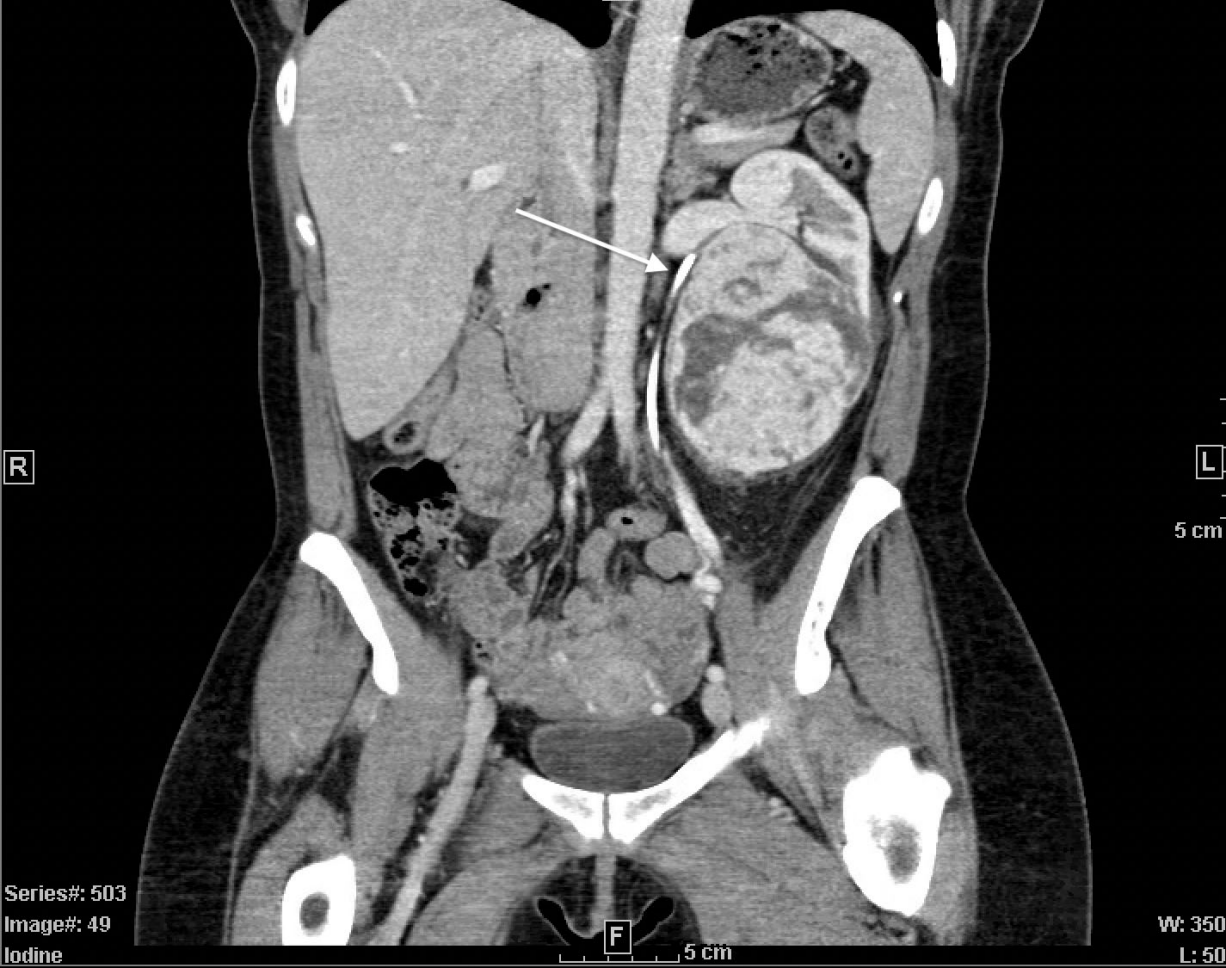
CT-Abdomen with IV contrast. Coronal view demonstrating large, 7.5x8.3x10.0cm heterogeneous solid mass in solitary left kidney. Stent in place *(white arrow)*, indicating compressed and arched ureter superior to the mass.
